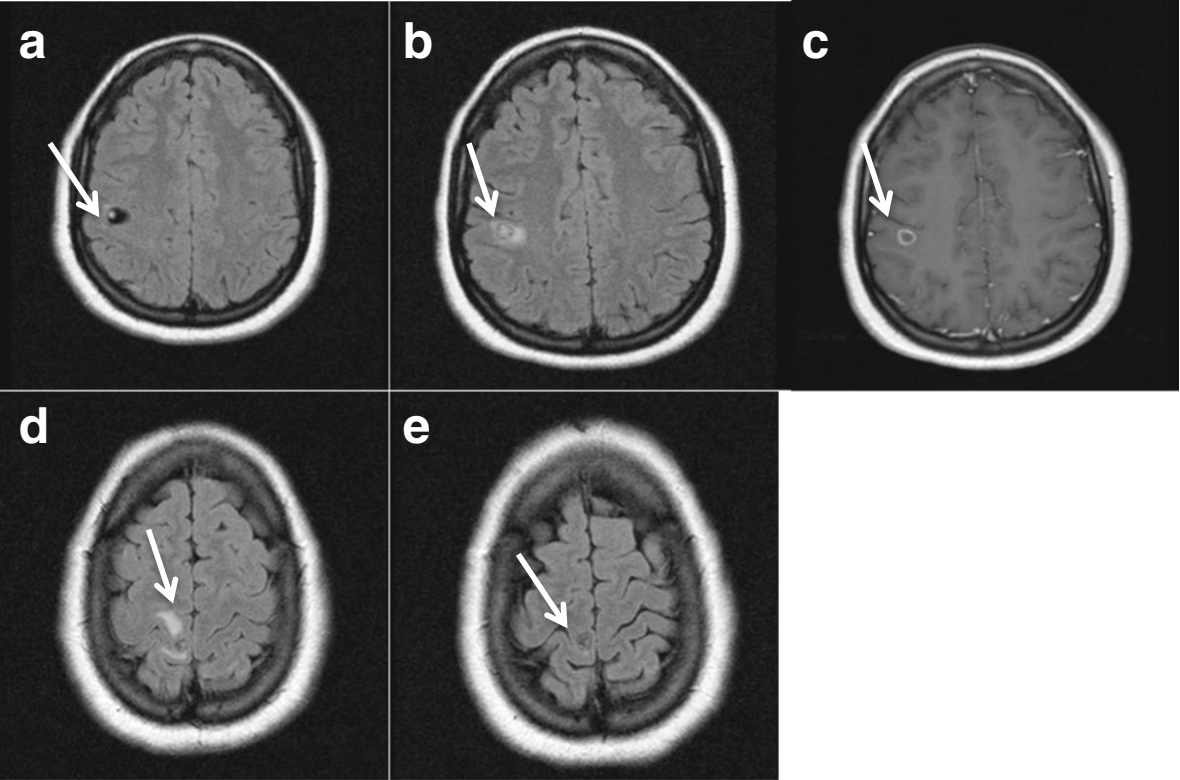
Brain MRI of patient 1 before and after treatment. a FLAIR sequence, cysticercus scolex at vesicular stage before treatment; b FLAIR sequence, scolex surrounded by edema after treatment; c T1 sequence with gadolinium after treatment, single enhancing lesion of scolex at vesicular stage; d FLAIR sequence, edema consistent with cysticercus cyst at colloidal stage before treatment, e FLAIR sequence, persisting lesion without edema after treatment
